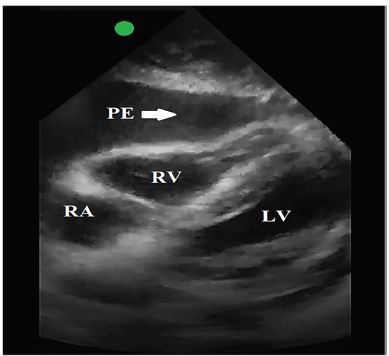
In this subxiphoid view obtained with a phased array, point-of-care ultrasound probe, there is obvious pericardial effusion with right ventricular wall collapse. This demonstrates pericardial tamponade physiology in combination with the patient’s vital signs and physical exam findings. *RV*, right ventricle; *LV*, left ventricle; *RA*, right atrium; *PE*, pericardial effusion.
